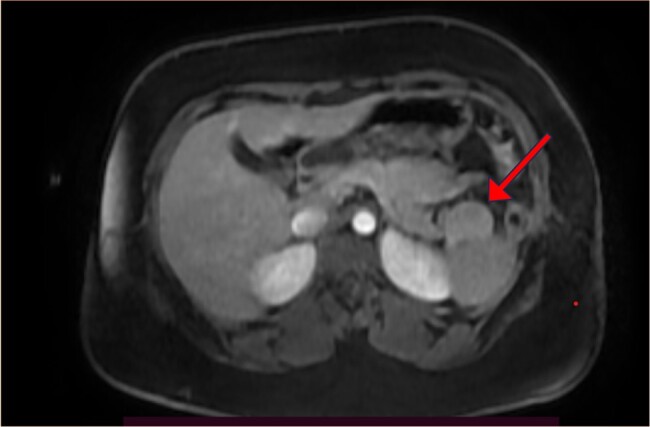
Axial section of the abdominal magnetic resonance imaging scan—an oval-shaped, well-marginated, T1-isointense to splenic parenchymal, T2-hyperintense mass lesion with no restricted diffusion in diffusion-weighted imaging/apparent diffusion coefficient images, measuring 3.64 × 3.72 × 2.63 cm in H × T × AP diameter.
